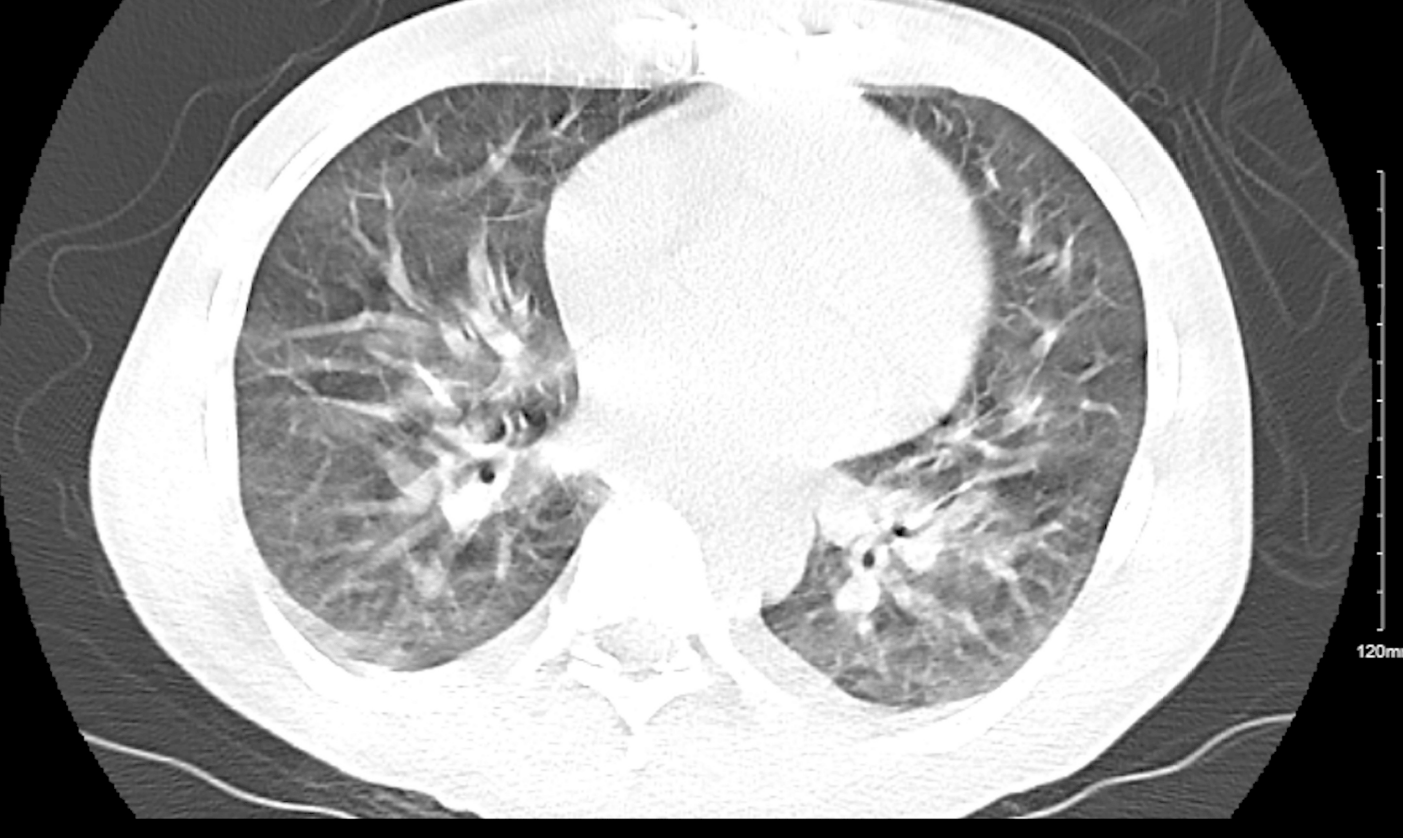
Chest CT scan (trans axial view, lung window) showing ground-glass opacities. CT: Computed tomography
